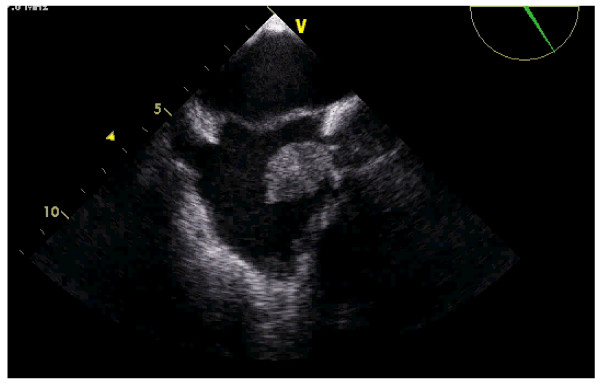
Transesophageal echocardiography. Bicaval modified view. The origin of the mass is in the superior vena cava, where the catheter may be seen.
